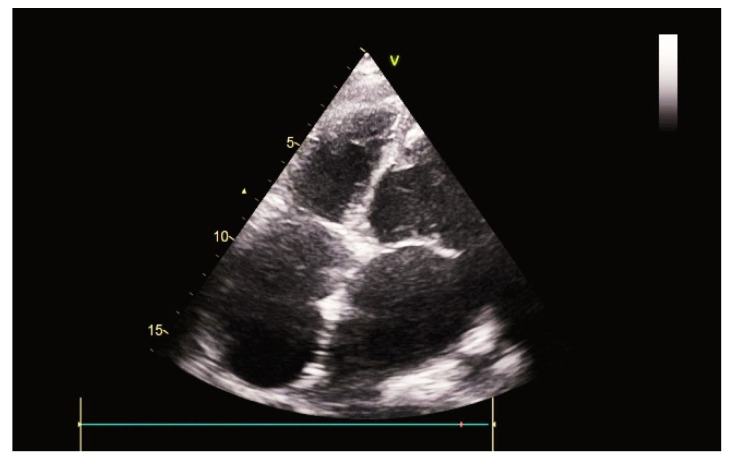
Ultrasound image after tRVAD explantation.
